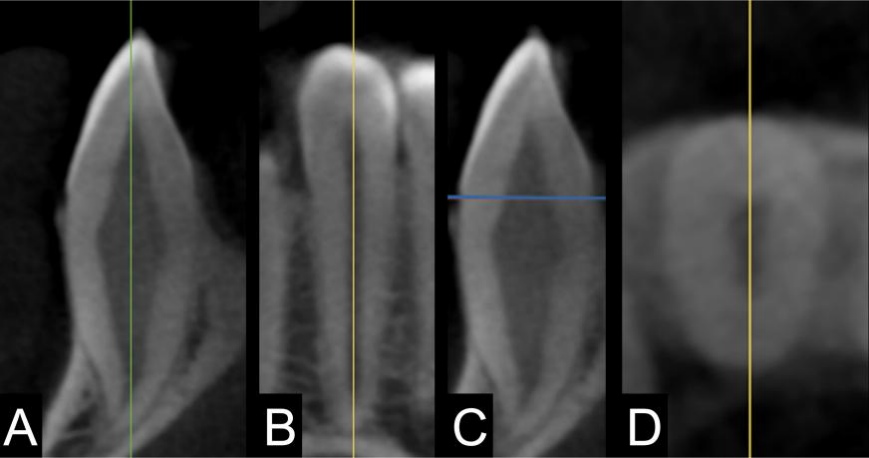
Representative cone-beam computed tomography cross sections: (A) sagittal and (B) coronal views highlighting the alignment of the CBCT volume with the longitudinal axis of the tooth; (C) sagittal view highlighting the determination of the cervical region and (D) the resulting axial view.
